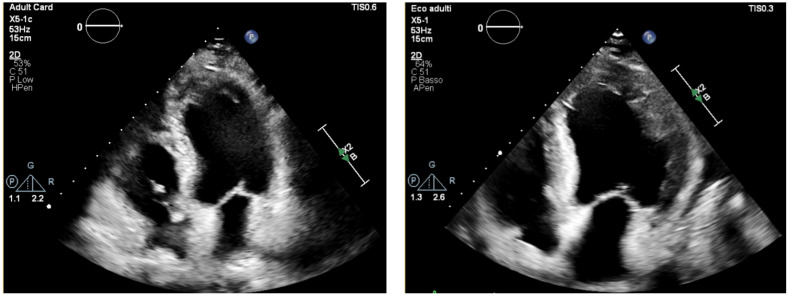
Transthoracic echocardiography, apical four-chamber view. Normal LV thickness and size (left), becoming pseudo-hypertrophic and hypokinetic after onset of eosinophilic myocarditis (right).
